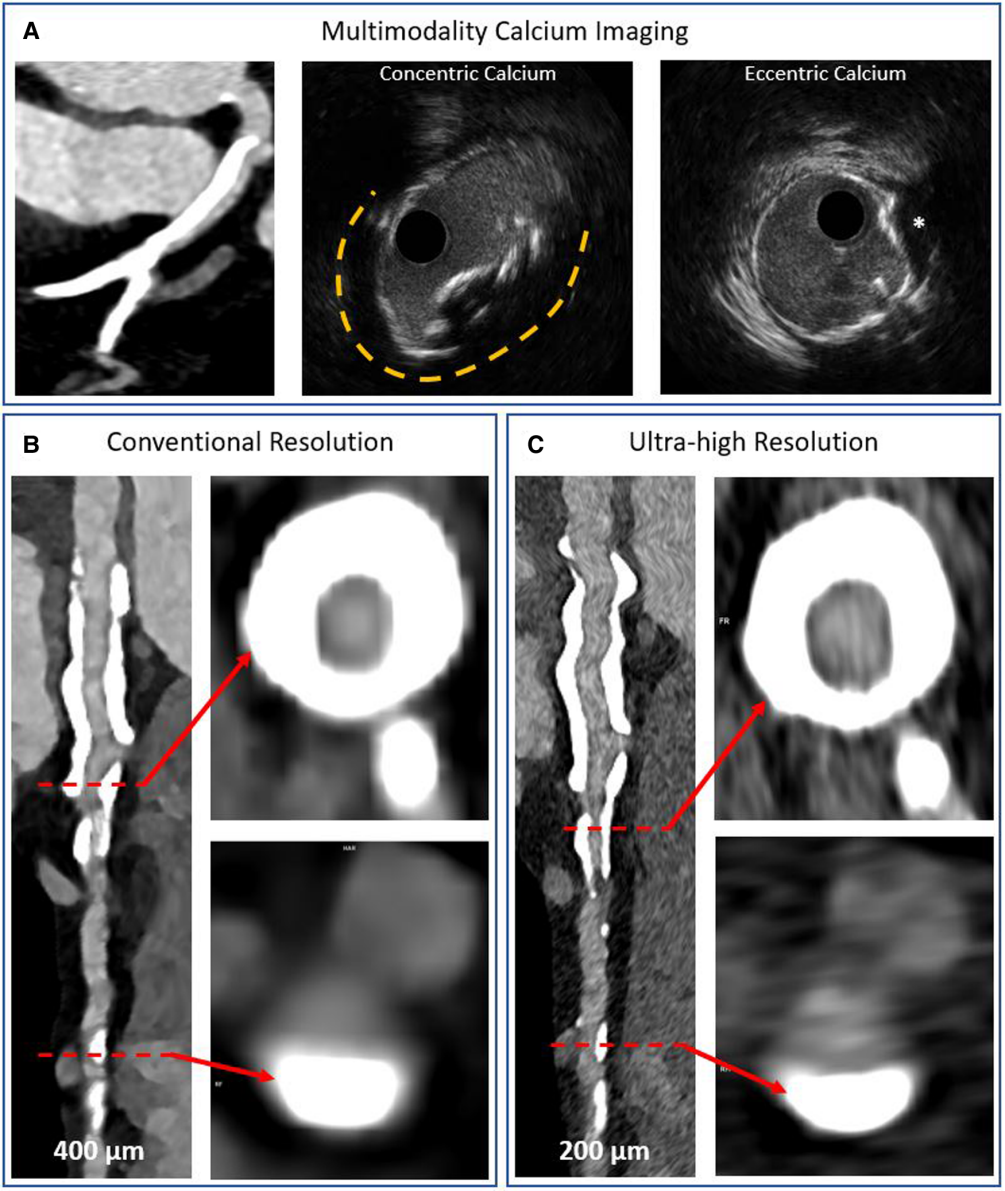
Multimodality imaging of calcified coronary arteries. Panel A shows a heavily calcified coronary artery segment on conventional detector CT imaging and intravascular ultrasound imaging examples of concentric and eccentric calcification patterns. The asterisk denotes a calcium nodule. Panels B,C show photon counting CT acquisitions of a heavily calcified vessel with concentric and eccentric calcification patterns at conventional and ultra-high resolutions, respectively. The blooming artefact is reduced while the lumen is more clearly visualized in the ultra-high resolution acquisition.
